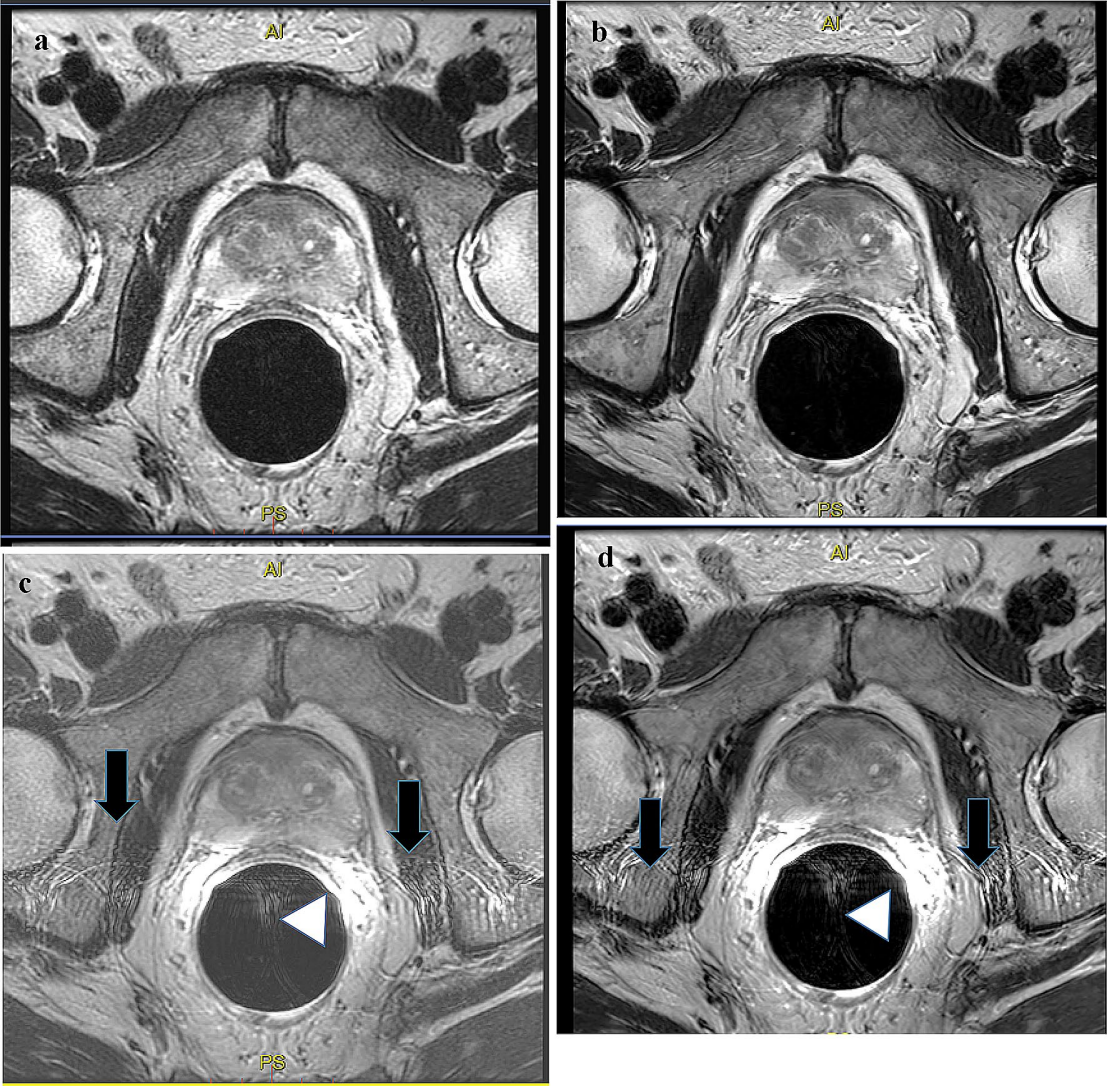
Axial T2-weighted images of a 61-year-old patient with a history of prostate cancer. a Images obtained with the ERC signal turned off and without DLR (Non-ERC_Conv_). b Images generated with the ERC signal turned off and with DLR (Non-ERC_DLR_). c Images obtained with the ERC signal turned on and without DLR (ERC_Conv_). d Images obtained with the ERC signal turned on and with DLR (ERC_DLR_). On c and d, note the pulsation artifacts from the coil (black arrows) and the near field artifacts (arrowhead) that are not seen on a or b
